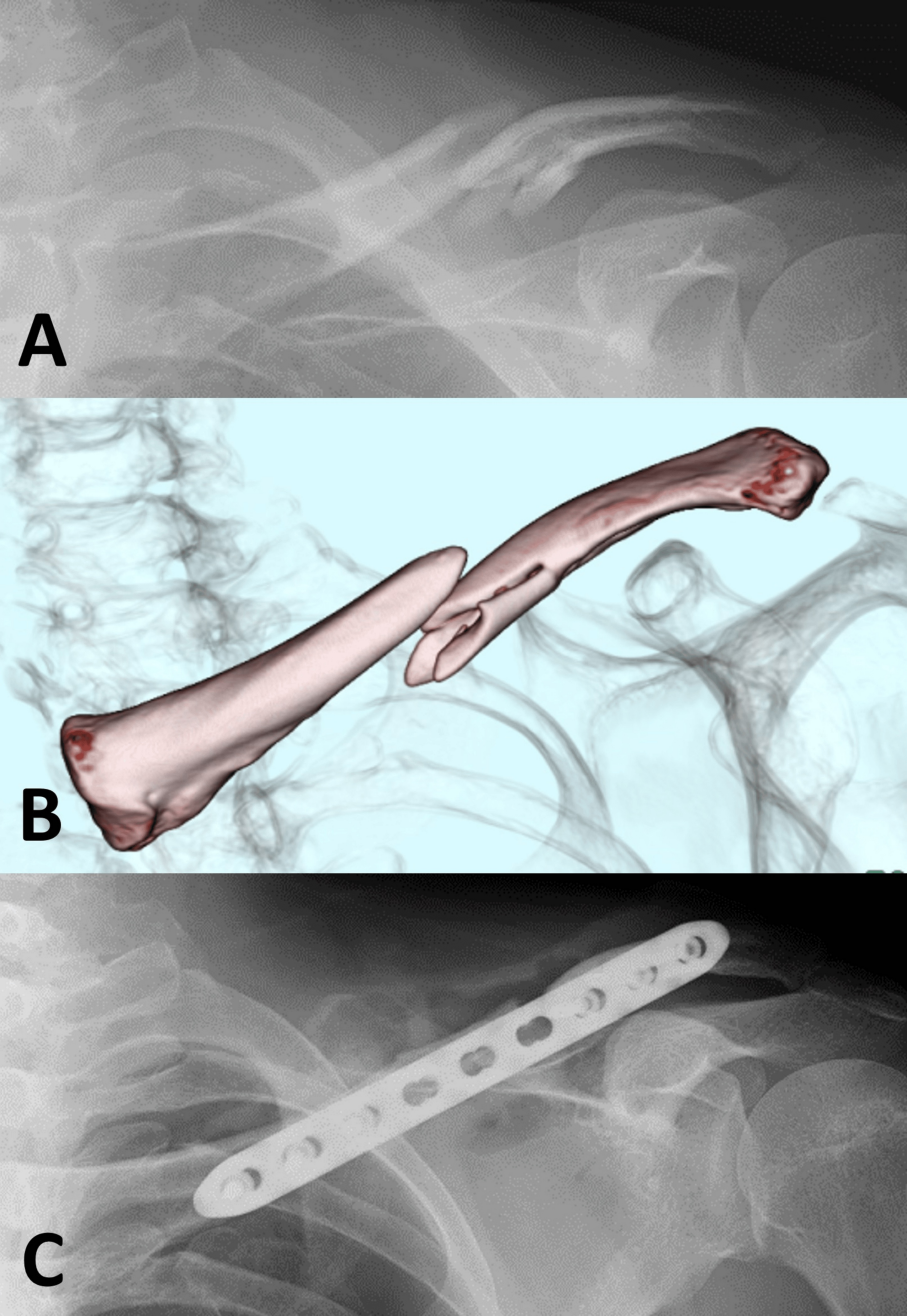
Injury and initial surgery X-ray imaging at the time of injury revealed a displaced midshaft fracture of the left clavicle (A), while three-dimensional computed tomography showed two fragments at the fracture site (B). Postoperative X-ray imaging showed anterior plate fixation using only cortical screws (C).
